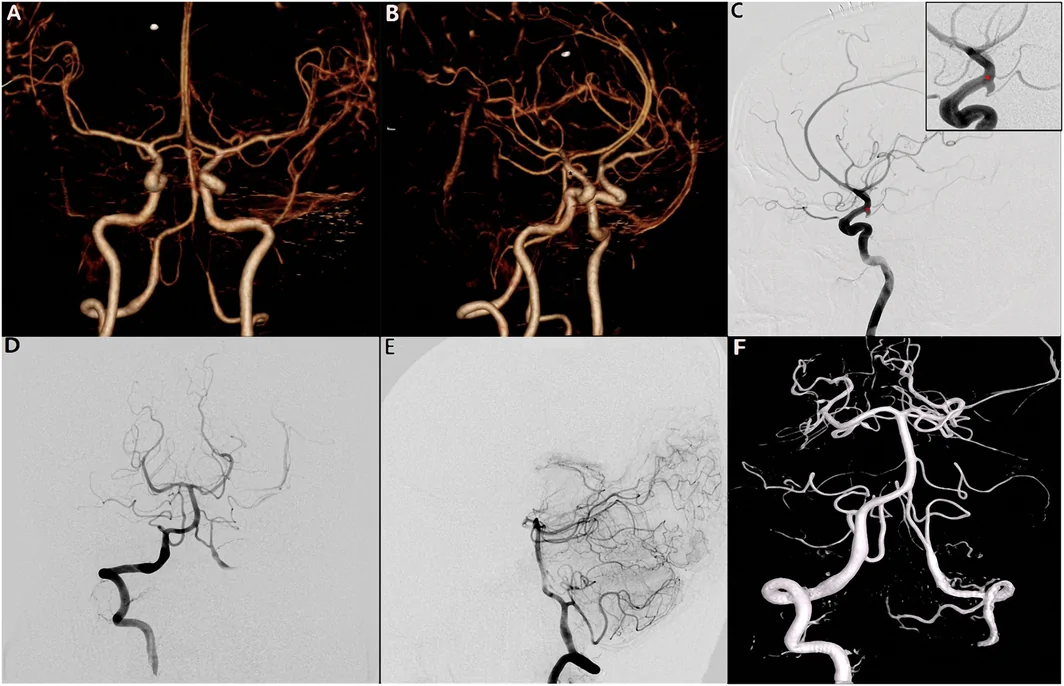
Neurovascular imaging findings after subarachnoid hemorrhage detection. (A) Computed tomography angiography did not identify a definite ruptured aneurysm or other causative vascular lesion. (B) Initial digital subtraction angiography showed no definite aneurysm, dissection, arteriovenous shunt, or other causative vascular abnormality. (C) A small vascular pouch measuring approximately 3 × 3 mm was noted at the right posterior communicating artery origin. The right posterior communicating artery arose from the apex of the pouch, and the lesion had an infundibular configuration without a discrete aneurysmal neck. The magnified inset in the upper right corner highlights the vascular pouch more clearly, supporting the multidisciplinary interpretation of junctional or infundibular dilatation rather than a definite ruptured aneurysm. (D) Right vertebral artery anteroposterior digital subtraction angiography without manual neck compression showed opacification of the contralateral left posterior inferior cerebellar artery. (E) Lateral view of right vertebral artery digital subtraction angiography. (F) Rotational three‐dimensional vertebral artery angiography showed no definite aneurysm, dissection, arteriovenous shunt, or other causative vascular abnormality.
